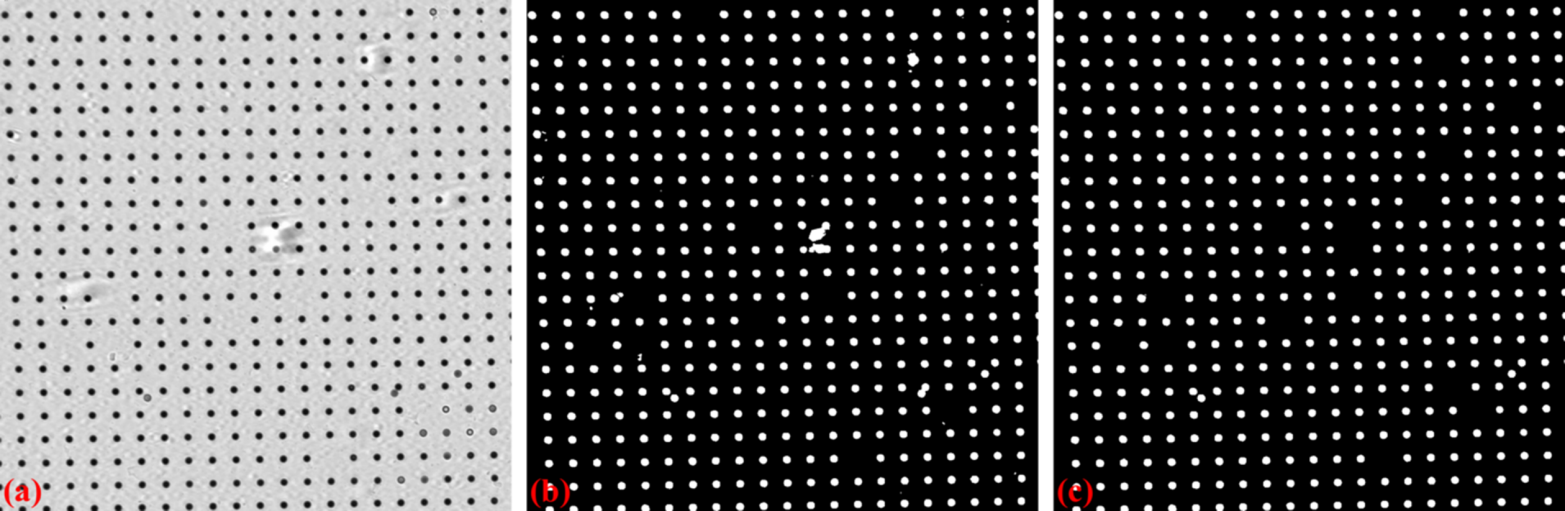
Demonstration of removing non-dot objects: (*a*) dot-pattern image (X-ray target), (*b*) binary image, (*c*) image with non-dot objects removed.
